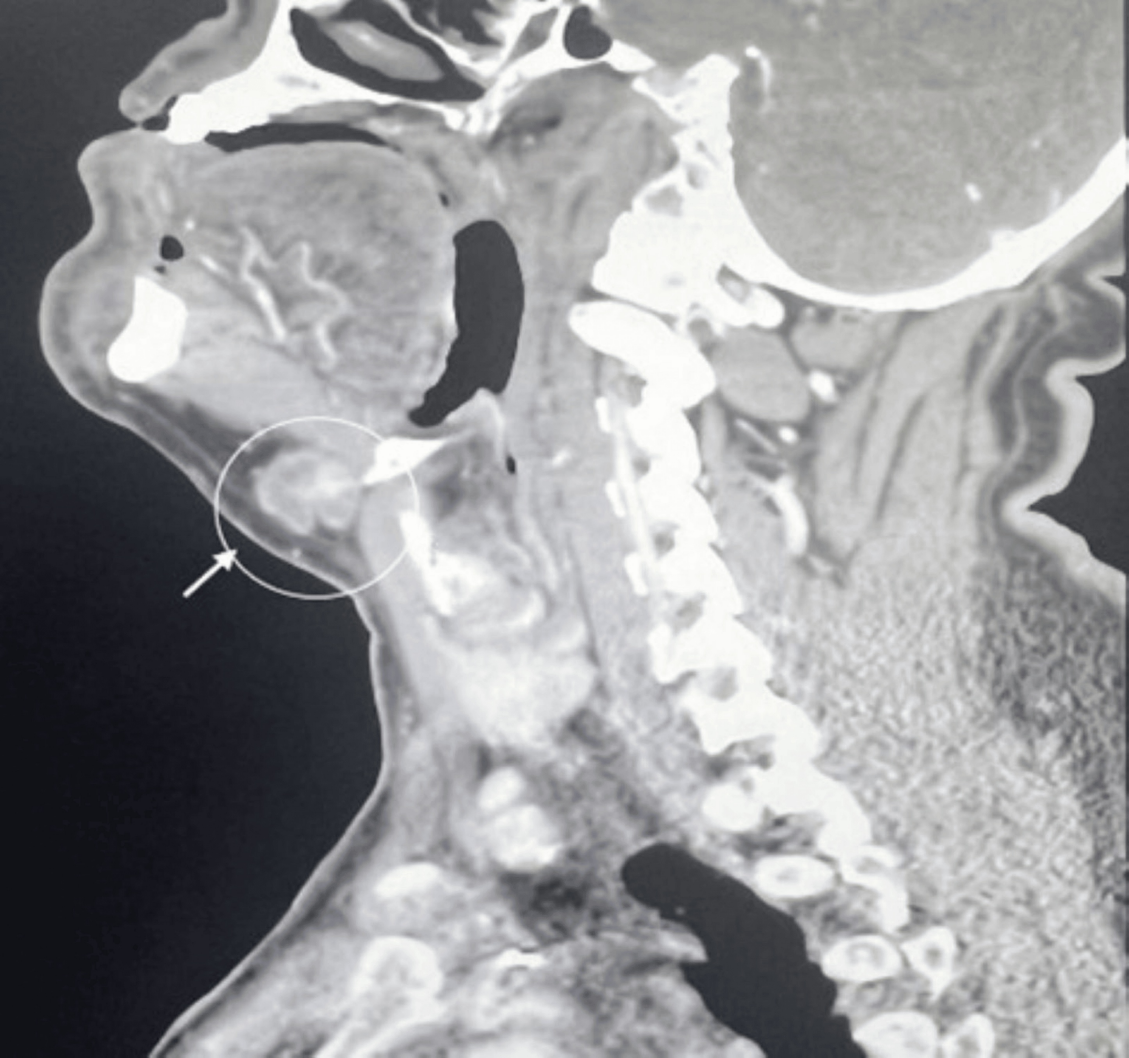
A contrast-enhanced sagittal CT scan of the hyoid bone level shows a multilocular cystic lesion in the midline of the neck, measuring 4 cm by 3 cm. The mass features a thin rim of contrast and contains fluid with a density that exerts pressure on the airway.
